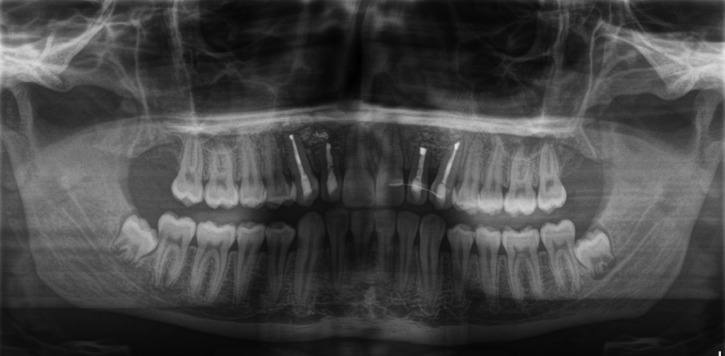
Panoramic X-ray after three months presenting the surgical site and the teeth which were involved in the procedures.
